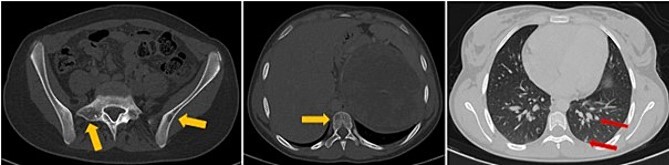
Axial thoraco-abdominal CT image on the bone window showing lytic bone lesions of the left iliac wing, sacrum, and vertebral bodies and on the pulmonary window showing pulmonary nodules.
